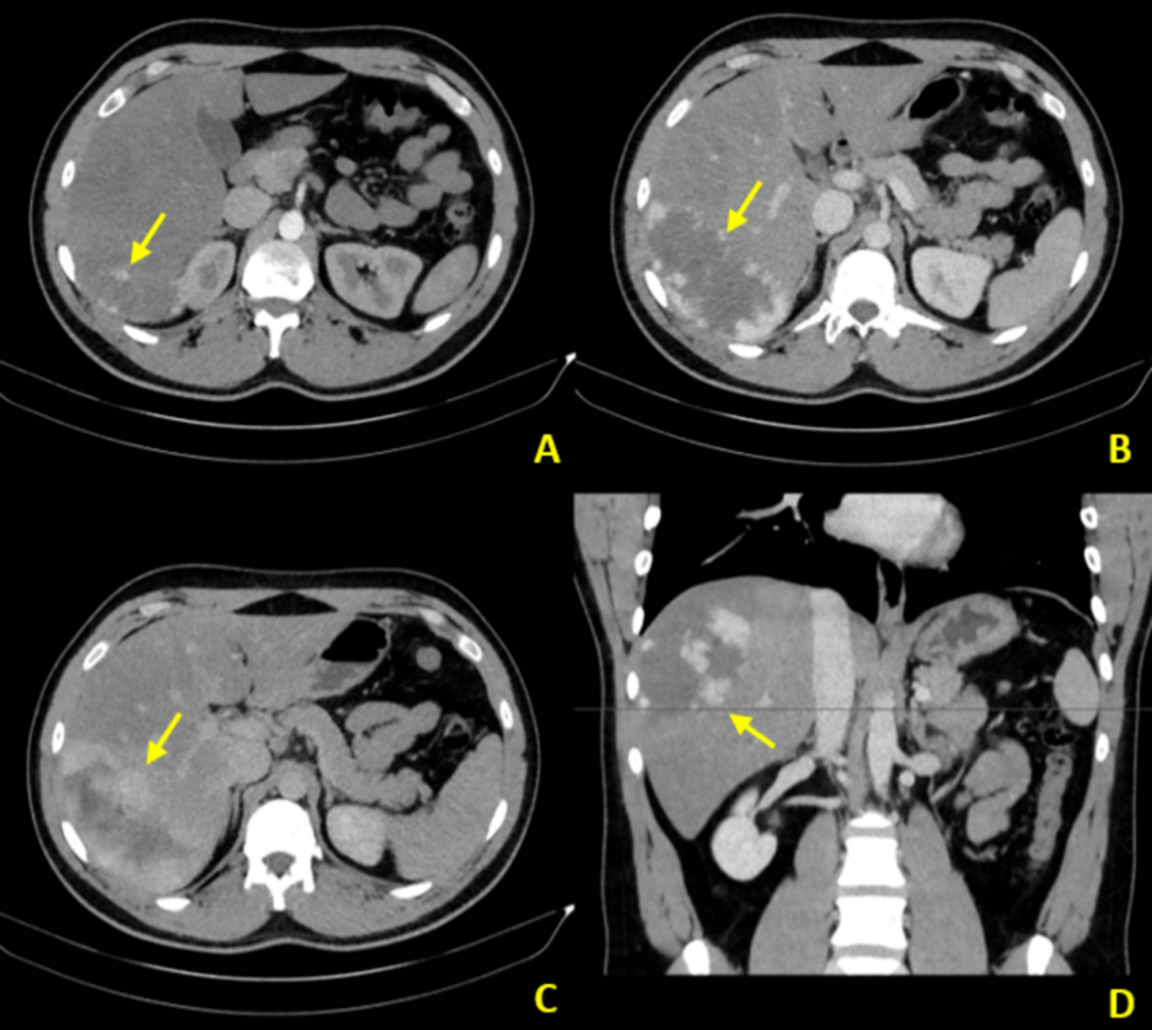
Triple-phase contrast-enhanced CT images CT: computed tomography A. Late arterial phase axial CT image in the same patient demonstrates discontinuous peripheral nodular enhancement (arrow) – classic for hepatic cavernous hemangiomas. B. Portal venous phase axial CT image at a more superior slice more clearly demonstrates the enhancement pattern (arrow). C. Venous phase axial CT image demonstrates slow centripetal filling (arrow). D. Portal venous phase coronal CT image provided to demonstrate location of hemangioma (arrow)
